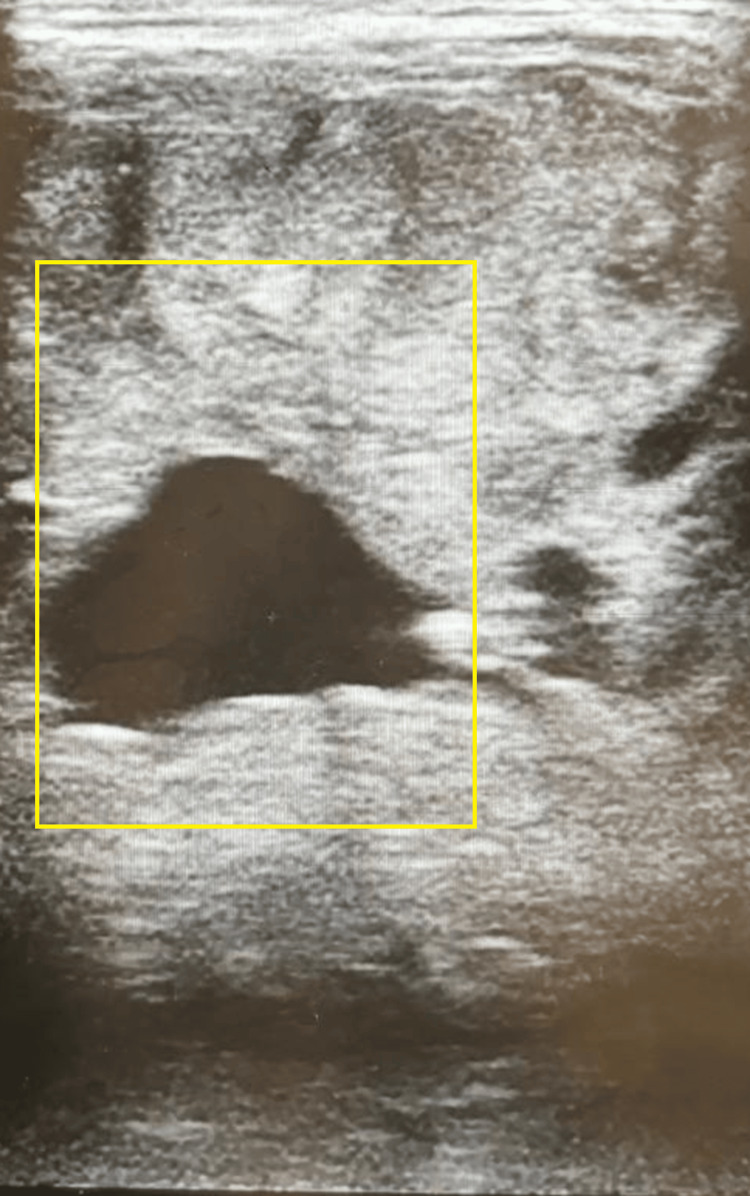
Ultrasonography of the neck showing anechoic cystic lesion with multiple separations
